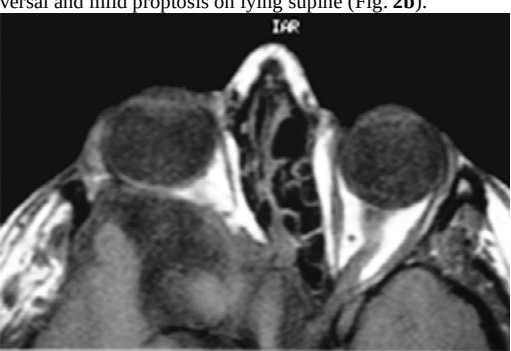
T1 weighted MRI scan showing right sided herniation of brain contents through the orbital bony structural abnormality with proptosis of the globe.
